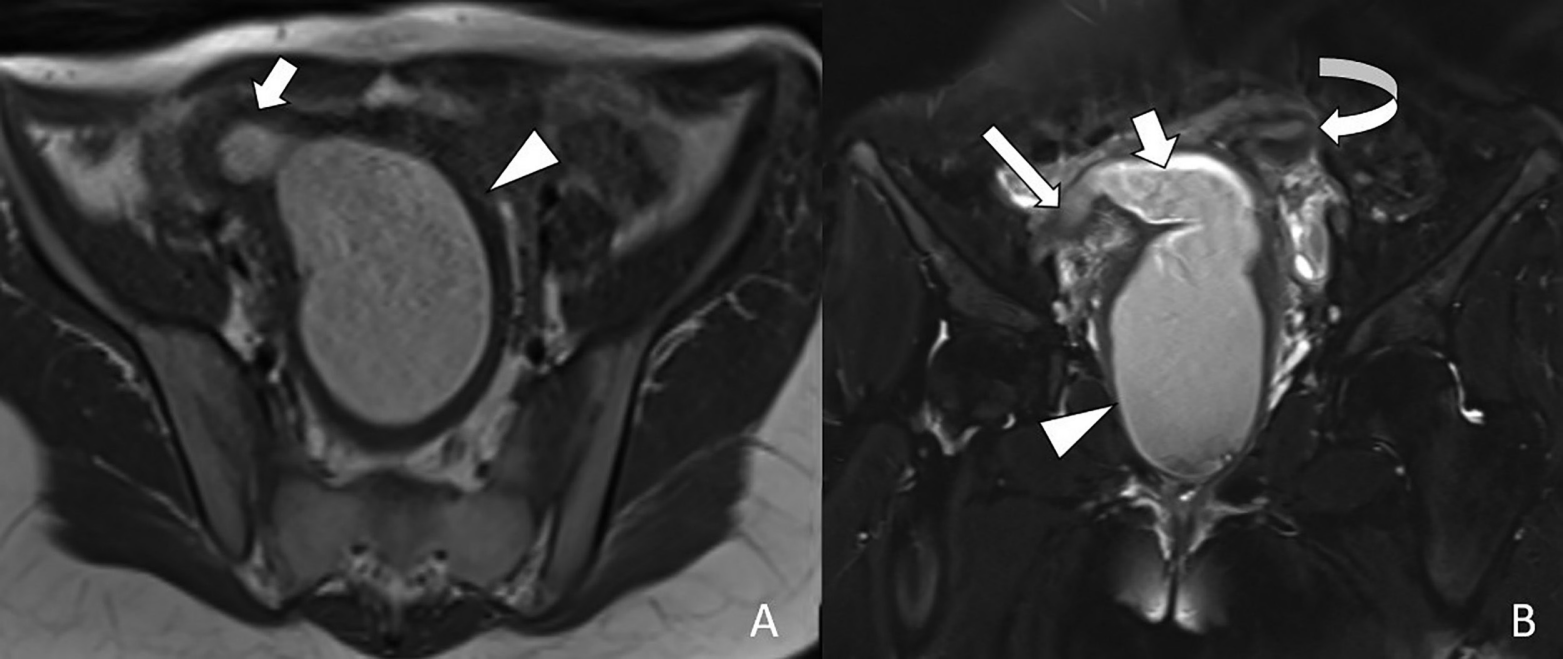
On unenhanced pelvic MRI, axial T1W series show hyperintense signal secondary to hemorrhagic material in the uterine cavity (the short arrow) and vagina (arrowhead) (A). Coronal plane images show a uterine didelphys appearance, and hemorrhagic material is observed in the right-sided uterine cavity (the regular arrow) and vagina (arrowhead). Also, hemorrhagic material continued in the right fallopian tube, causing expansion (the long arrow). The left-sided uterine cavity is normal (the curved arrow), and the vagina is compressed (B).
